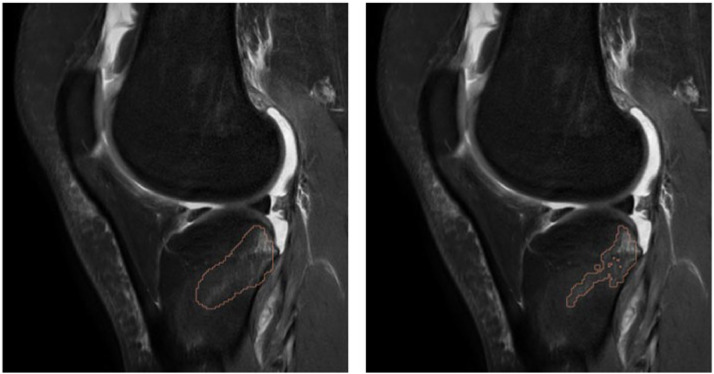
Retrospective study to evaluate the impact of artificial intelligence assistance on the diagnostic accuracy of radiologists for detecting bone marrow edema in knee MRI: Detection and segmentation of bone marrow edema by Keros software.
